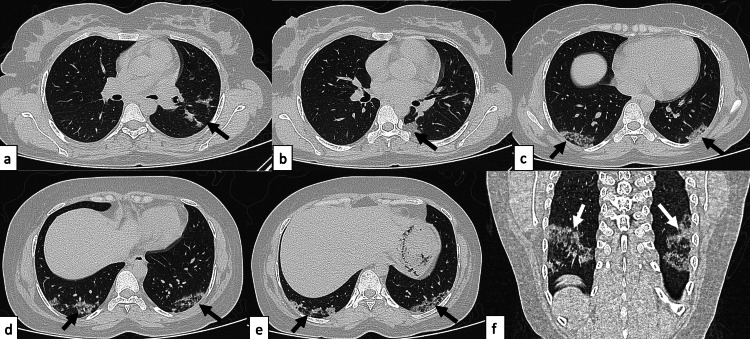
Organising pneumonia A 24-year-old female patient with SLE presented with complaints of dry cough of one-month duration. Axial (a-e) and coronal (f) HRCT chest images in the lung window revealed patchy areas of consolidation predominantly in subpleural locations (black arrows) noted in the bilateral lower lobes with a perilobular pattern of distribution, showing a reverse halo sign (white arrows) suggestive of organising pneumonia. SLE: systemic lupus erythematosus; HRCT: high-resolution CT
